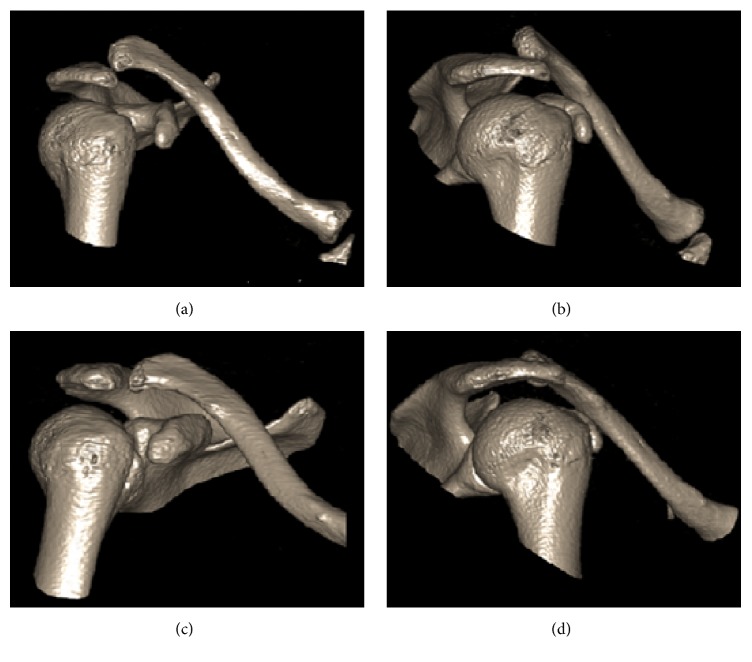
3D-CT scan after the patient created a dislocation ((a) and (b)) and after the patient created a comfortable situation ((c) and (d)).
